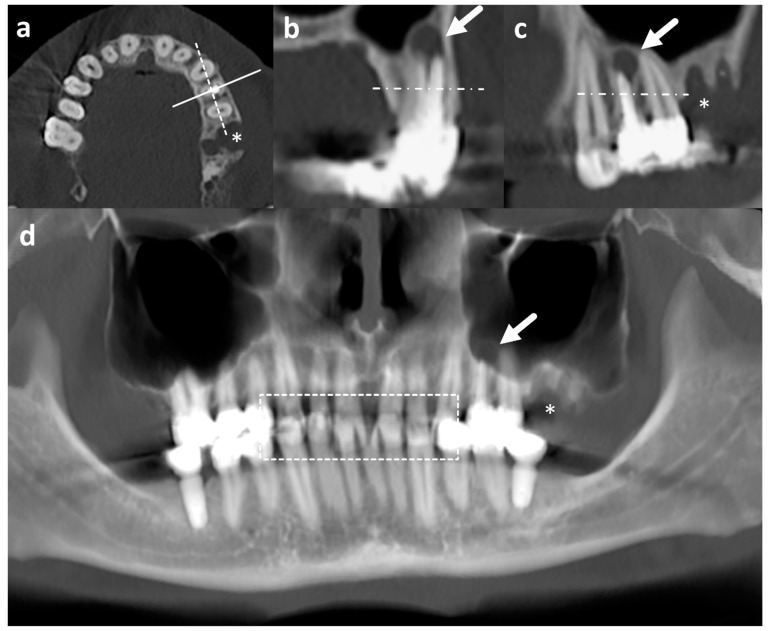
Dental part of CT images in axial (a), sagittal (b), and coronal plane (c), and vOPG (d) of the same patient. The solid and dashed line in a corresponds to the plane of sagittal (b) and coronal (c) reconstructions reconstructed in 1 mm slice thickness, respectively. Note the periapical abscess at the left maxillary first premolar with root canal treatment (arrow in (b–d)) is much more conspicuous on CT than vOPG. The asterisk (*) marks a defect after tooth extraction (a,c,d). The dashed box in d contains areas of overlap due to closed jaws during examination impairing evaluability particularly of vOPG. Horizontal streak artefacts may be noted in all reconstructions parallel to the image acquisition plane in all reconstruction resulting from metal implants. CT = computed tomography examination; vOPG = virtual orthopantomogram.
